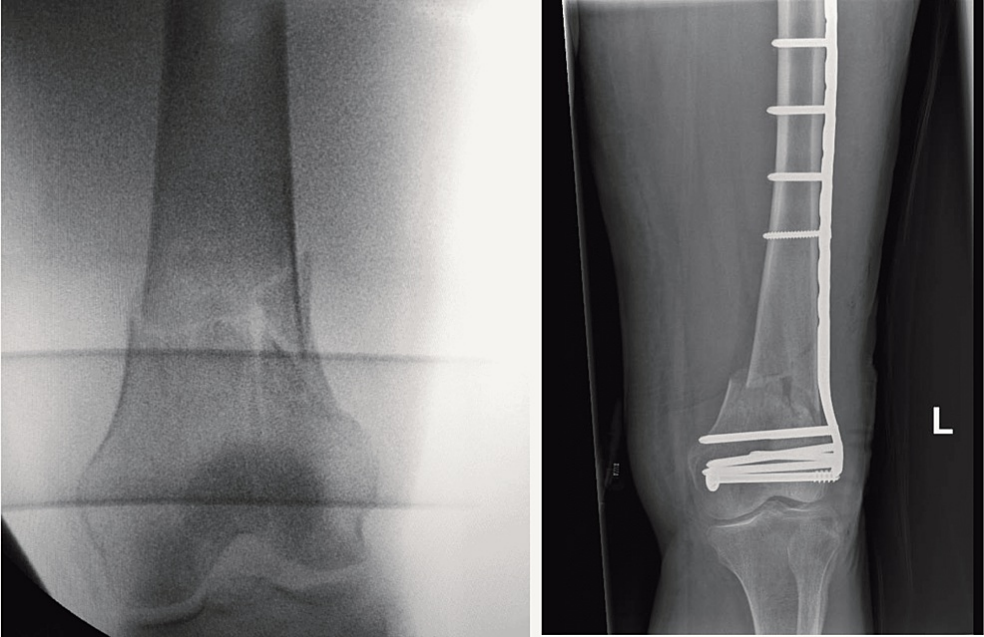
Intraoperative radiographs with the described setup taken by the image intensifier showing before (left) and after fixation (right).
